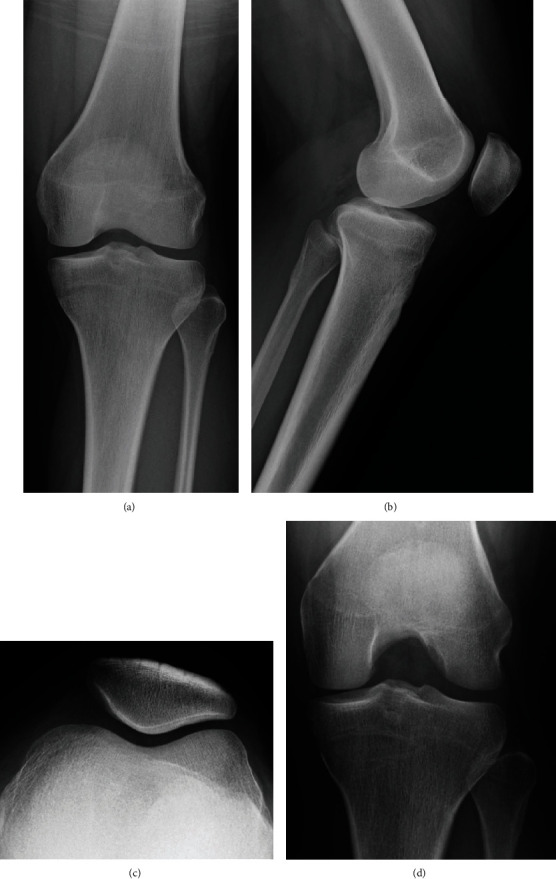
Preoperative radiography. The preoperative radiography was normal. (a) Anteroposterior view; (b) lateral view; (c) skyline view; (d) Rosenberg view.
